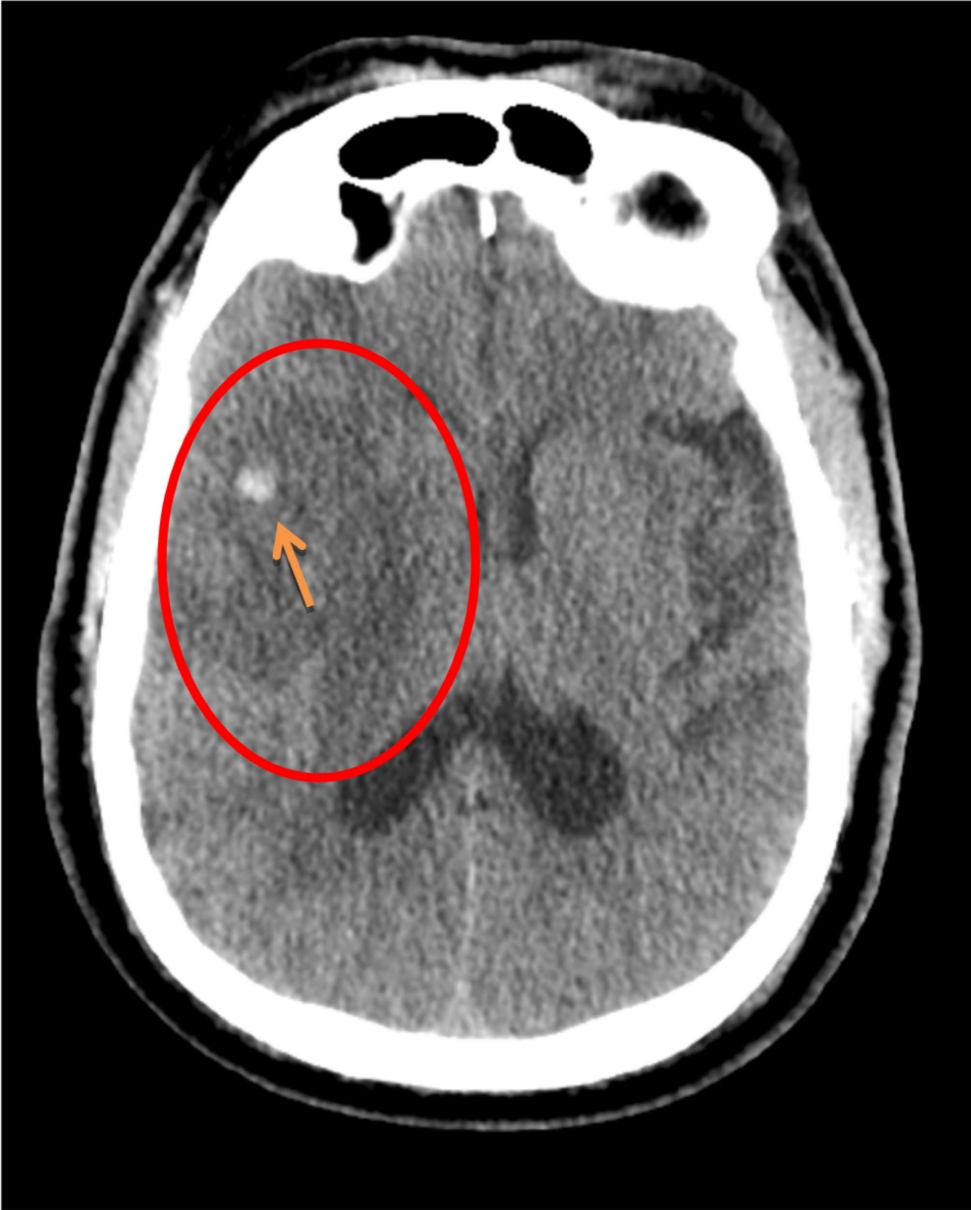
Noncontrast brain CT demonstrating large hypodensity corresponding to right MCA infarct (red circle). Within the area of infarct is a small hemorrhagic focus (orange arrow)
